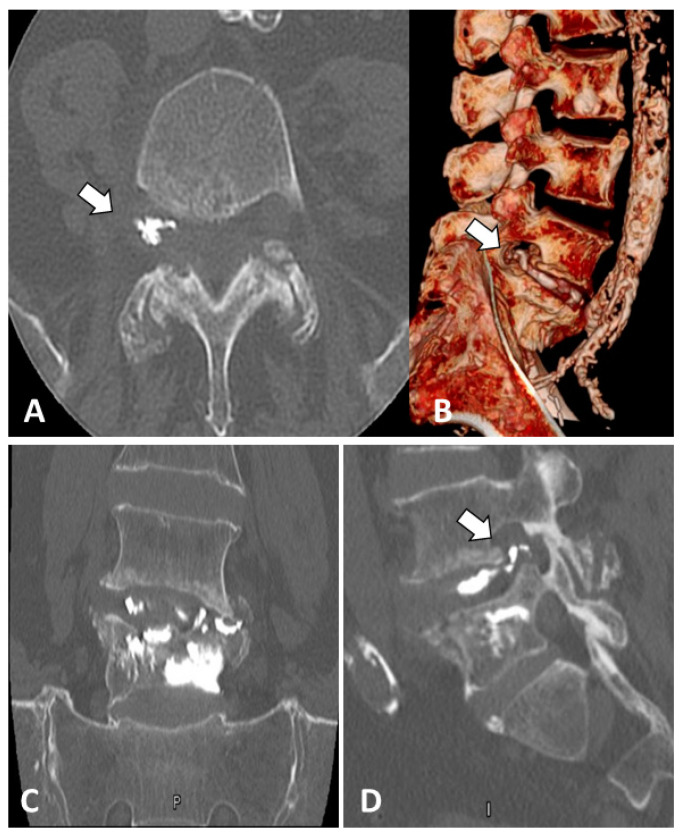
(A) Axial CT scan at the fracture level, documenting cement leakage in the neuroforamen (white arrow); (B) A 3D CT reconstruction of the lumbar spine: cement extravasation visible in the right neuroforamen (white arrow); (C) sagittal CT scan of the L5 fracture after VP; (D) sagittal CT scan at the fracture level, documenting cement leakage in the neuroforamen (white arrow).
